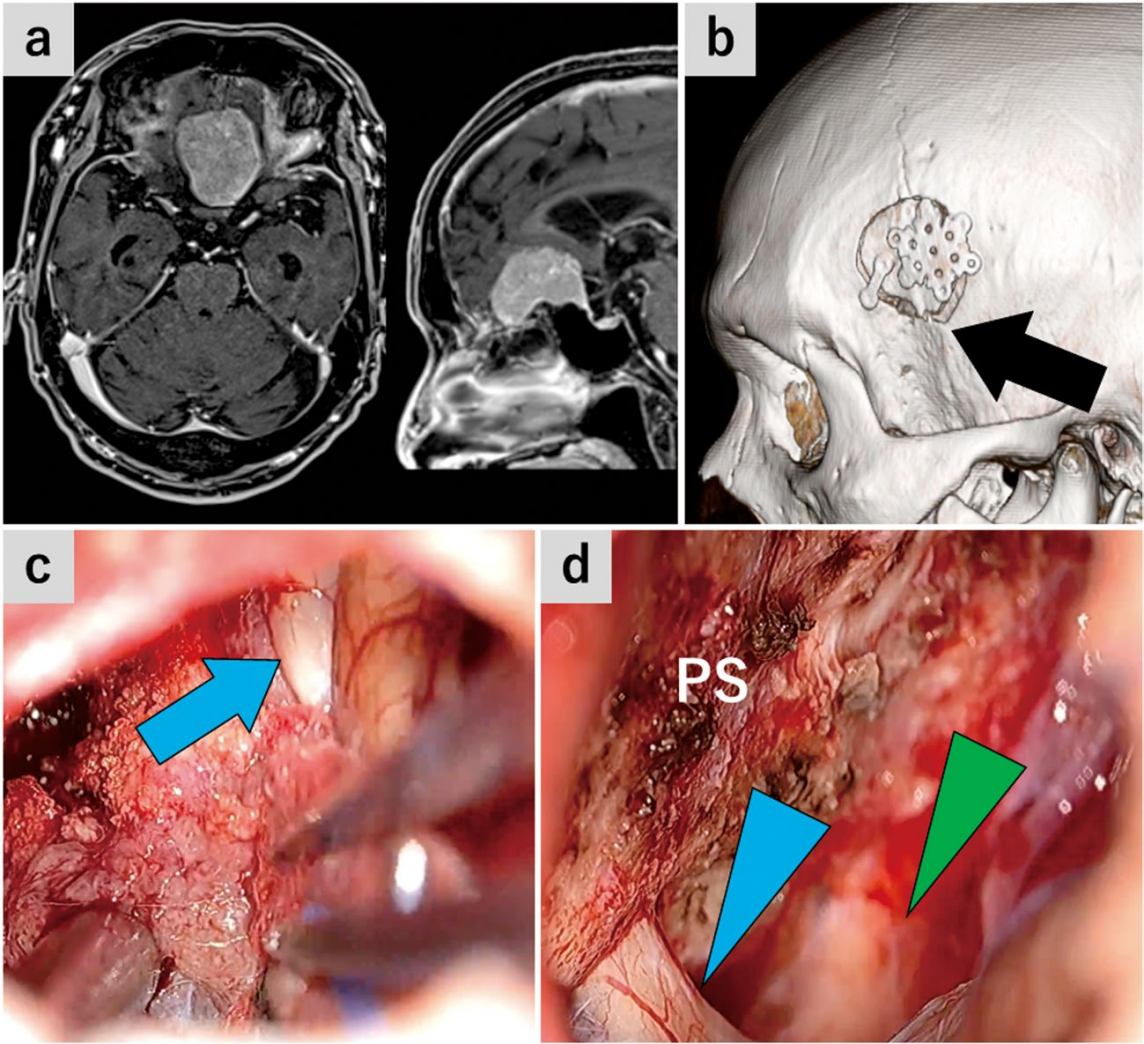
Case 1: Planum sphenoidale meningioma (a) Preoperative gadolinium-enhanced T1-weighted magnetic resonance image (MRI). b Postoperative 3D computed tomography shows craniotomy. Black arrow, ICP. (c and d) The tumor’s posterior part was exoscopically removed. c The posterior part of the tumor was separated from the bilateral olfactory tracts and frontal lobule. Blue arrows, left olfactory tract. (d) The posterior part of the tumor attaching to the planum sphenoidale was removed. Blue arrowhead, left optic nerve; green arrowhead, right optic nerve; PS, planum sphenoidale
